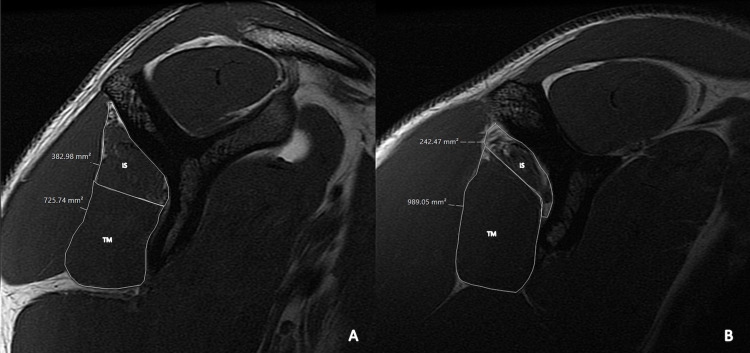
Sagittal T1-weighted MRI of the right shoulder with the area calculated for teres minor (TM) and infraspinatus (IS) from 2019 (A) and 2021 (B).
